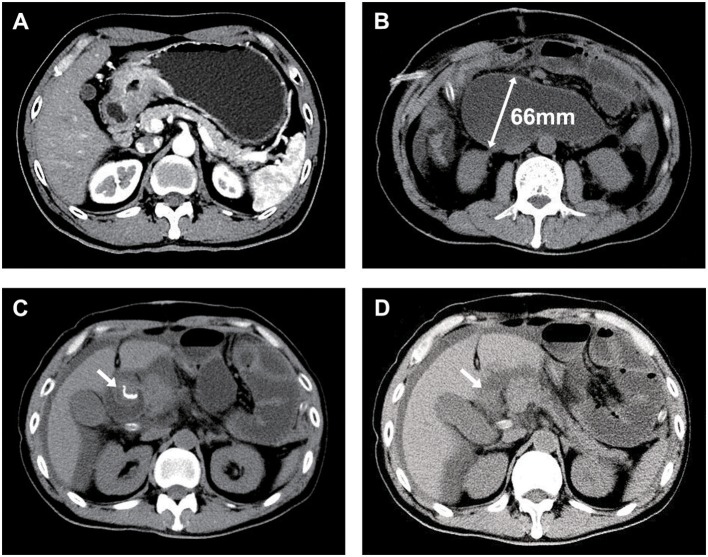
One case of afferent loop obstruction after Billroth II reconstruction and single purse-string suture for reinforcement of duodenal stump. (A) Abdominal CT image of the case with adenocarcinoma of gastric antrum; (B) After the operation, the afferent loop obstruction occurred, and the diameter of duodenum was more than 6 cm; (C,D) The reinforced duodenal stump (arrows) was intact.
